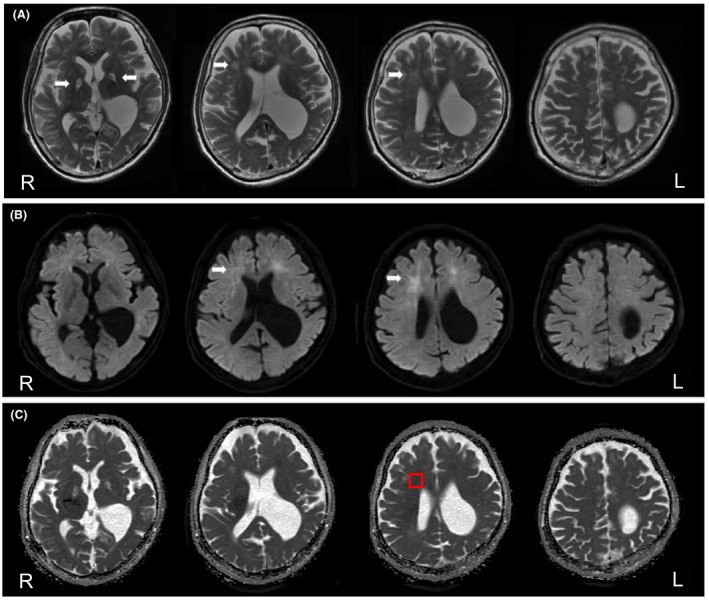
Magnetic resonance imaging (MRI) of the brain 26 days after the hypoxic insult. (A) T2 images reveal confluent hyperintense regions in the bilateral frontal white matter and the globus pallidus (arrows). (B) Diffusion‐weighted imaging (DWI) reveals hyperintensity regions in the white matter corresponding to the changes seen in the T2 images (arrows). (C) An apparent diffusion coefficient (ADC) map reveals no obvious hypointensity regions in the white matter corresponding to the changes seen in the T2 images and DWI.
